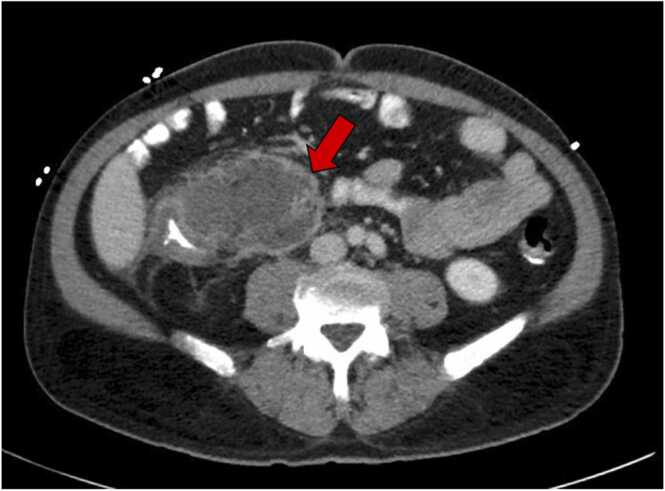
Repeat CT abdomen/pelvis on hospital day 9. Red arrow indicates a large 8.4 cm complex collection with surrounding mesenteric edema in the right lower quadrant is contiguous with the cecum, ascending colon, and terminal ileum.
